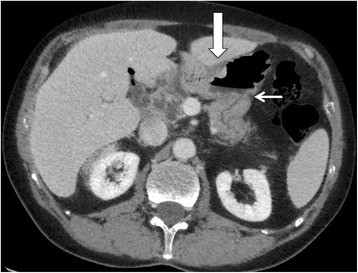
CT scan with contrast injection: axial view. The gastric wall is thickened (large arrow) in front of the pancreaticogastrostomy (thin arrow)
